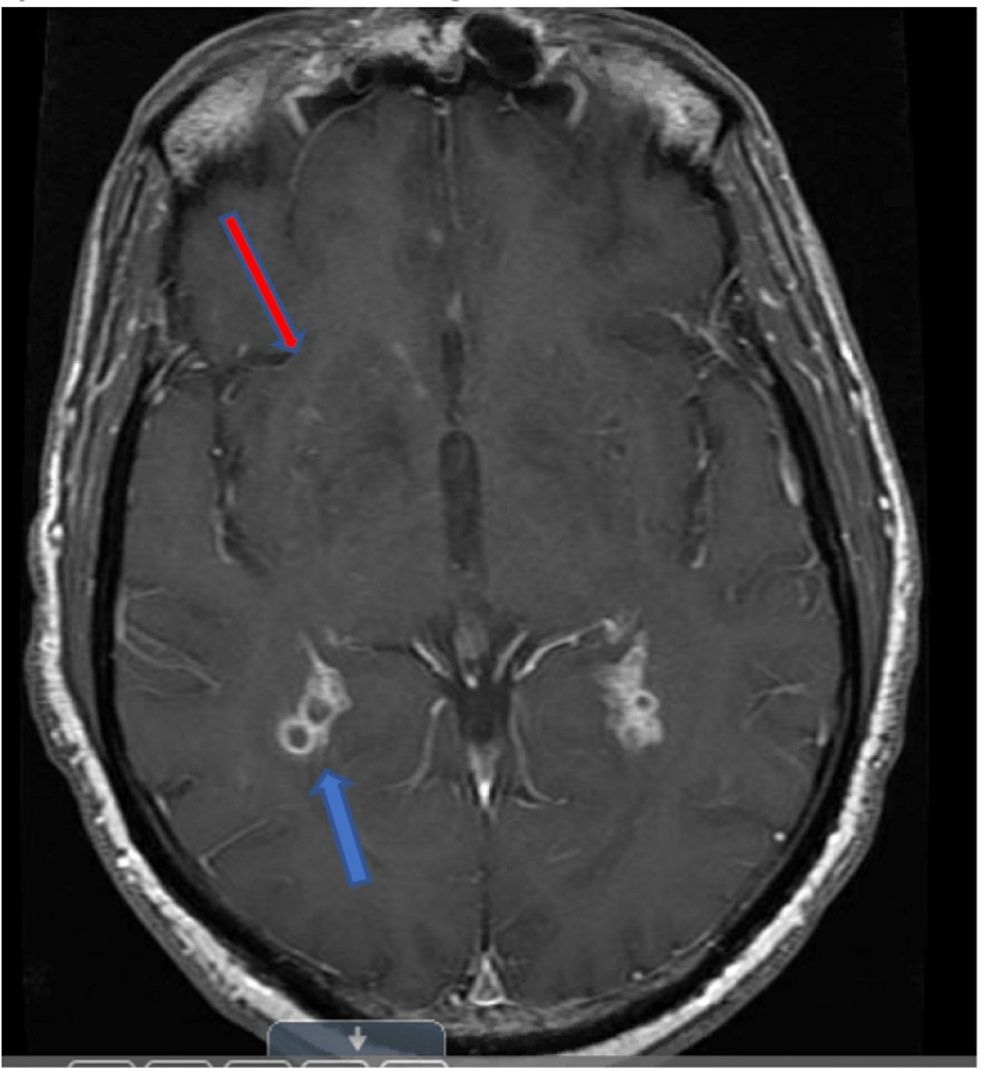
Contrast-enhanced magnetic resonance imaging shows perivascular enhancement in right basal ganglia (red arrow) and prominent enhancement of choroid plexus (blue arrow).
